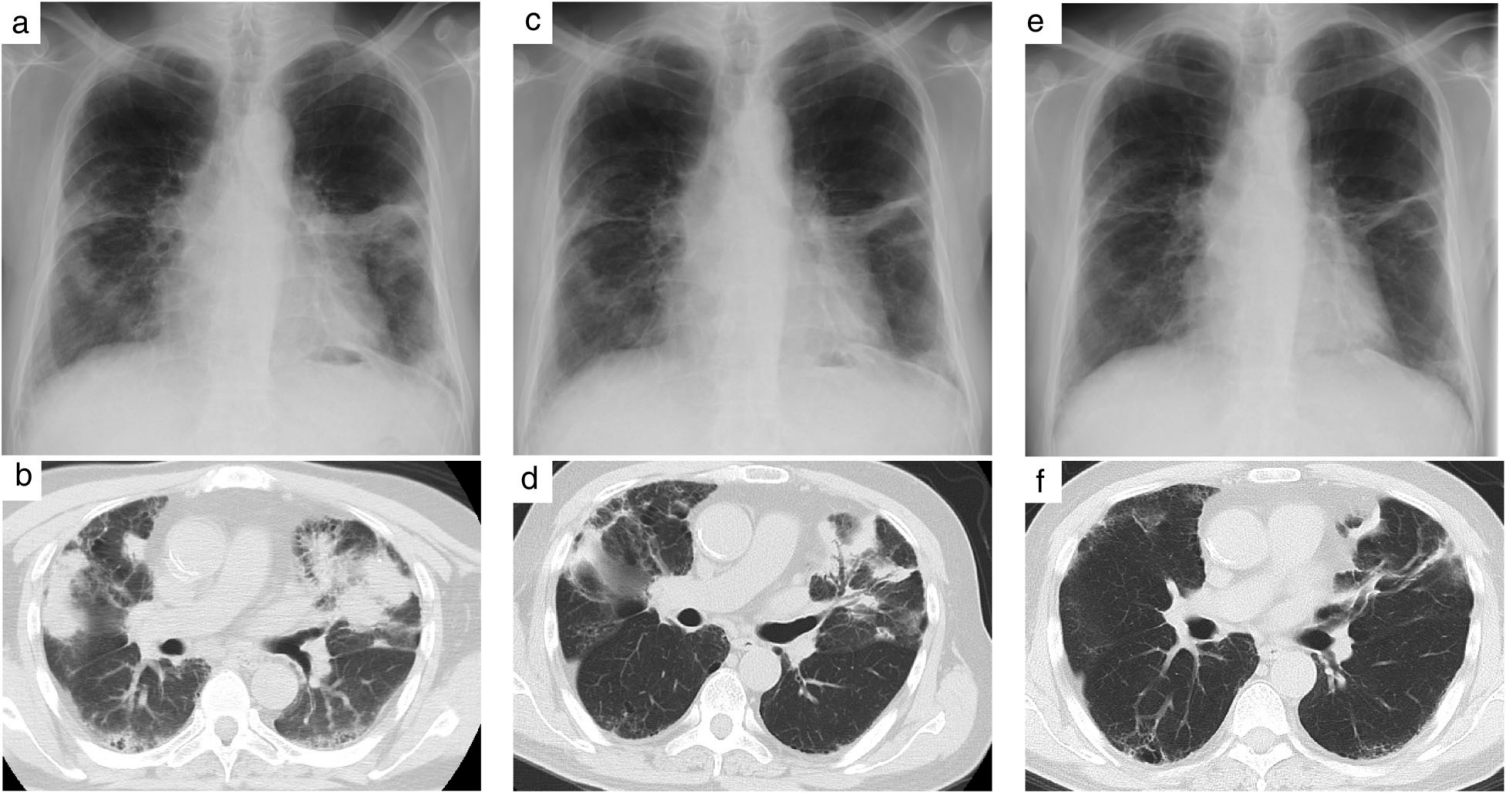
Interval changes of clinical images after chemotherapy. (a, b) Chest radiography and chest CT are performed just before the induction of chemotherapy. (c, d) Clinical images after two cycles of chemotherapy. (e, f) Clinical images after four cycles of chemotherapy
